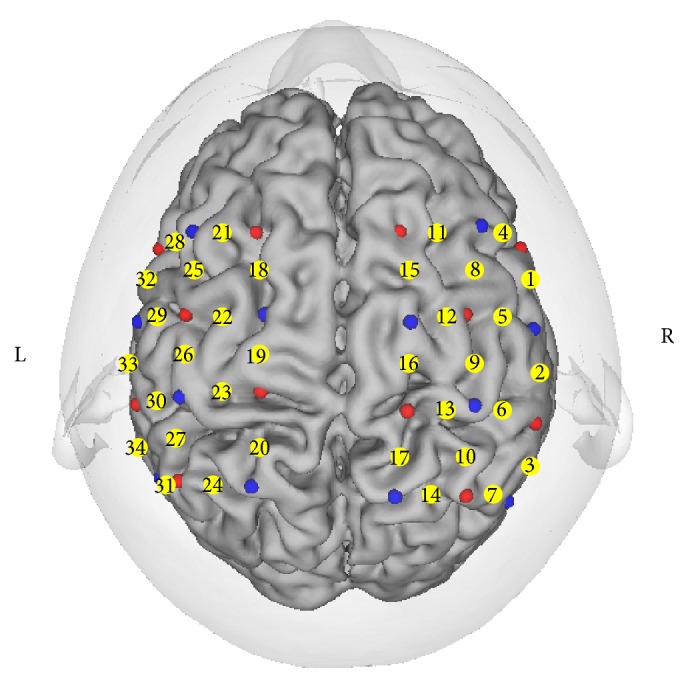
Projection of the probes and the channels onto the brain surface, using MRI data and a 3D position detector. Red: source, blue: detector, and yellow: channel.
